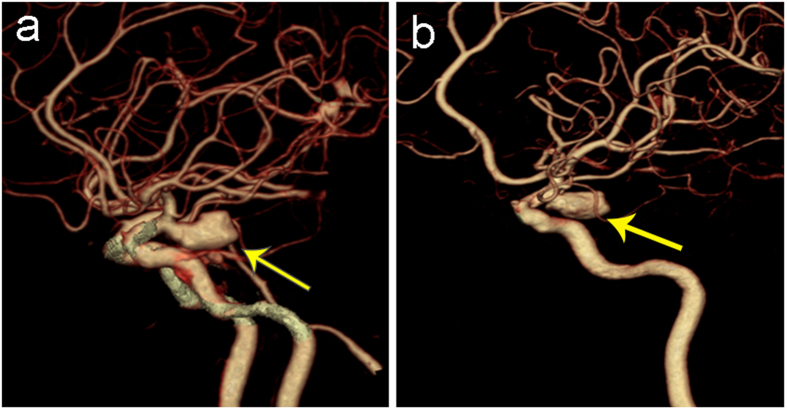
A 55-year-old women with a giant aneurysm in left posterior communicating artery. (a) Volume-rendered dual-energy CTA image shows a true-positive aneurysm (arrow) in the posterior communicating artery, which was confirmed by 3D-DSA (b).
